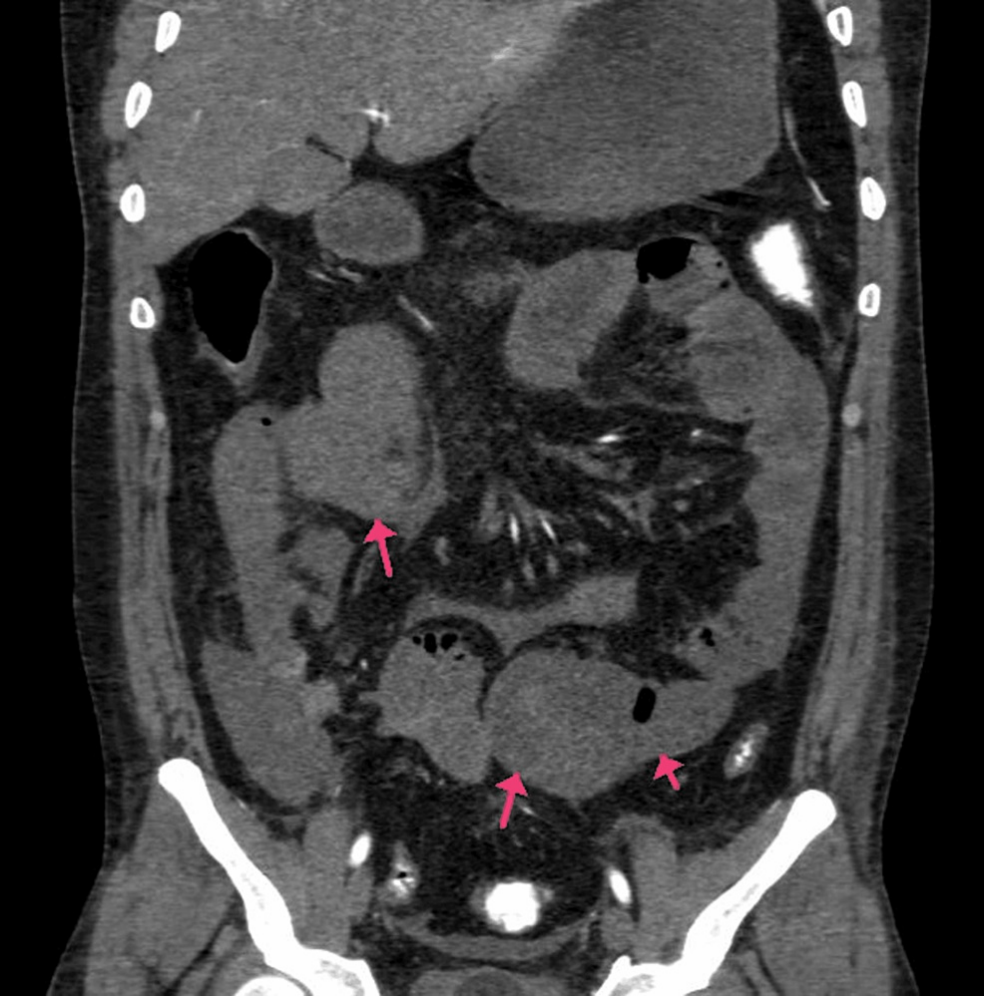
CECT of the abdomen and pelvis with angiography of the patient (arrows mild hypo-enhancement of the walls with a mildly dilated caliber of ileal loops and pneumatosis). CECT, contrast-enhanced computed tomography; SMA, superior mesenteric artery
